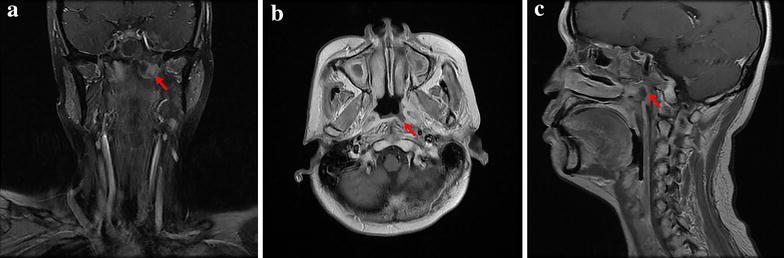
Magnetic resonance imaging of nasopharyngeal necrosis. a Coronal, contrast-enhanced, T1-weighted image shows non-enhanced soft tissues (like a hole) mixed with tiny air bubbles in the left nasopharyngeal lateral recess (*arrow*). b Transverse, contrast-enhanced, T1-weighted image shows the necrotic extent in the nasopharyngeal posterior wall (*arrow*). c Sagittal, contrast-enhanced, T1-weighted image shows an obvious defect in the left nasopharyngeal lateral recess (*arrow*)
